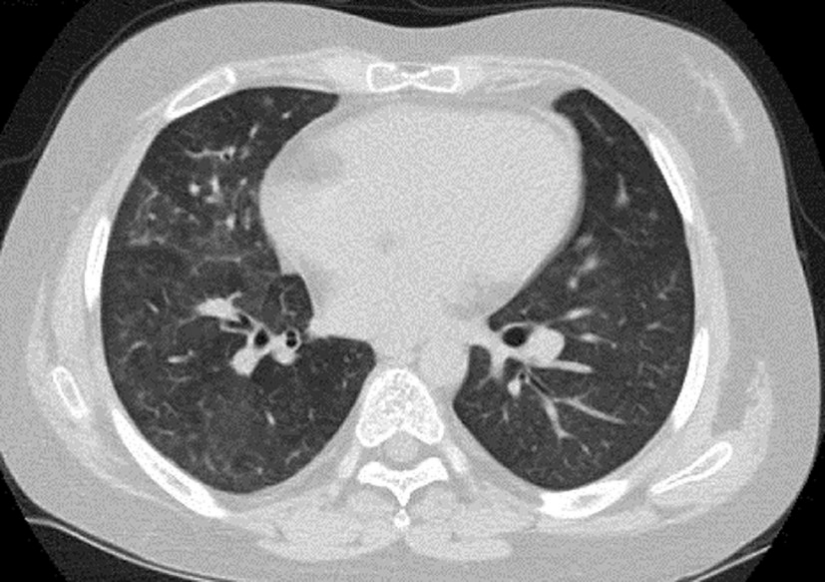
Post-transplant obstructive bronchiolitis. A 15-year-old girl with thalassemia who developed exertional dyspnea 18 months after hematopoietic stem cell transplantation. CT showed a mosaic perfusion pattern or admixture of hyperinflation and patchy GGOs of both lungs, associated with mild bronchial wall thickening
